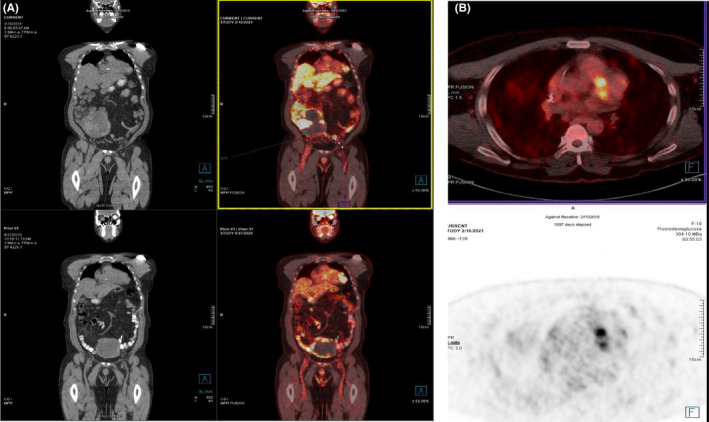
(A) PET CT coronal view showing nodules in the myocardium and mesentery along with a large abdominopelvic mass. (B) PET CT axial view showing nodules in the myocardium
